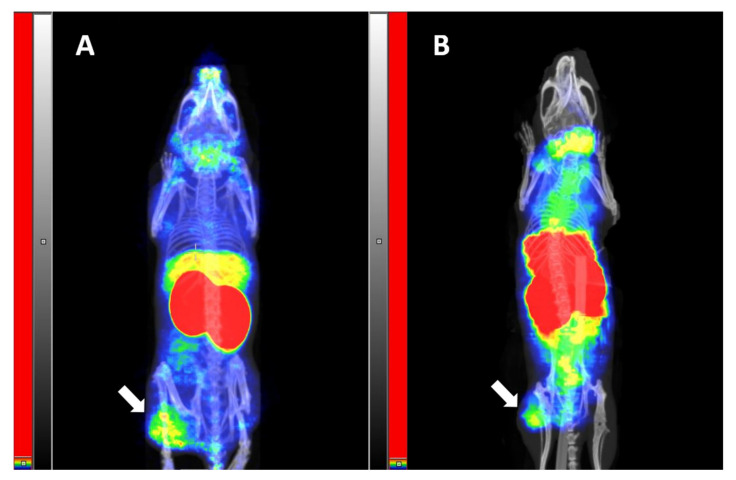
Imaging of IGF-1R expression in DU145 xenografts, using [^68^Ga]Ga-NODAGA-(HE)_3_-Z_IGF-1R:4551_ (A) and [^111^In]In-NODAGA-(HE)_3_-Z_IGF-1R:4551_ (B). The images were acquired 1 h after injection, using nanoScan PET/CT (A) and nanoScan SPECT/CT (B), and presented as maximum intensity projections. The arrows point at the tumors.
